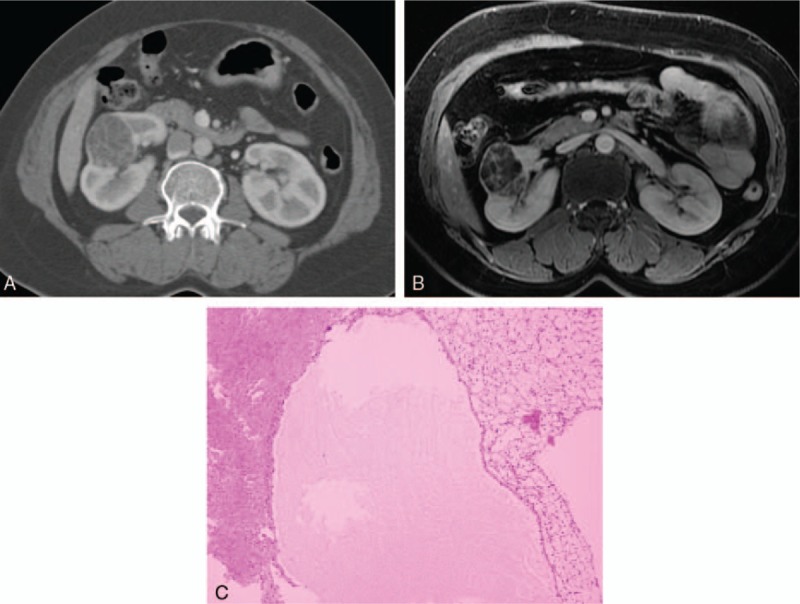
Images in a 45-year-old woman with a cystic mass in the right kidney. (A) Contrast-enhanced CT scan showed a complex cystic mass that contained a grossly thickened and enhancing septa, was consistent with a category III cyst. (B) Coronal Gadolinium-enhanced fat-suppressed T1-weighted MR image demonstrated more septa than are seen on the CT scan, and enhanced mural nodules were depicted on the wall/septa, caused the lesions to be upgraded to category IV. (C) This lesion was surgically removed and determined to be a renal cell carcinoma. CT = computed tomography.
